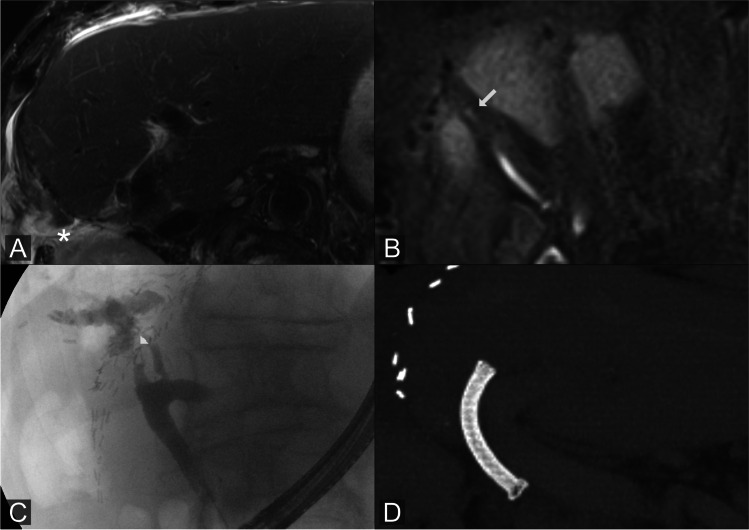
Peripheral bile leakage: MRI of a 70-year-old patient with colorectal liver metastasis after right hemihepatectomy. T2w image shows diffuse perihepatic fluid (asterisk) without formation of a biloma (A). Gd-EOB-DTPA-MRCP reveals a small extraluminal contrast agent collection at the surgical margin originating from the right hepatic duct (B). ERCP confirms the peripheral leakage at the resection margin (C). The patient was treated by endoscopic biliary stenting; the intraluminal metal stent covering the leakage site is visualized in this post-interventional CT maximum intensity projection (MIP) (D)
